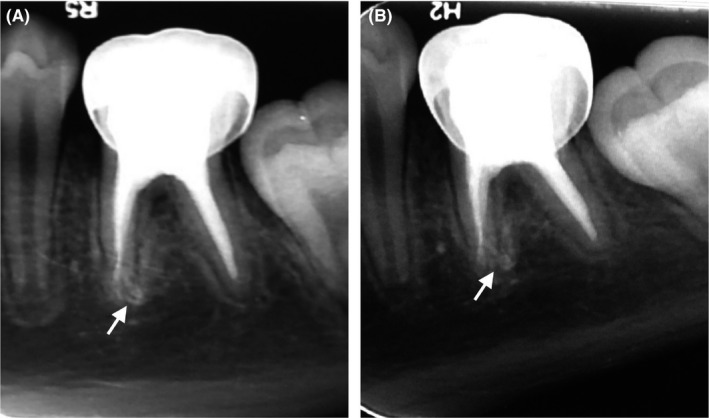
Follow‐up radiographs at (A) 16 and (B) 24 months. Resolution of the periradicular radiolucency and continued root development (arrows) are observed
